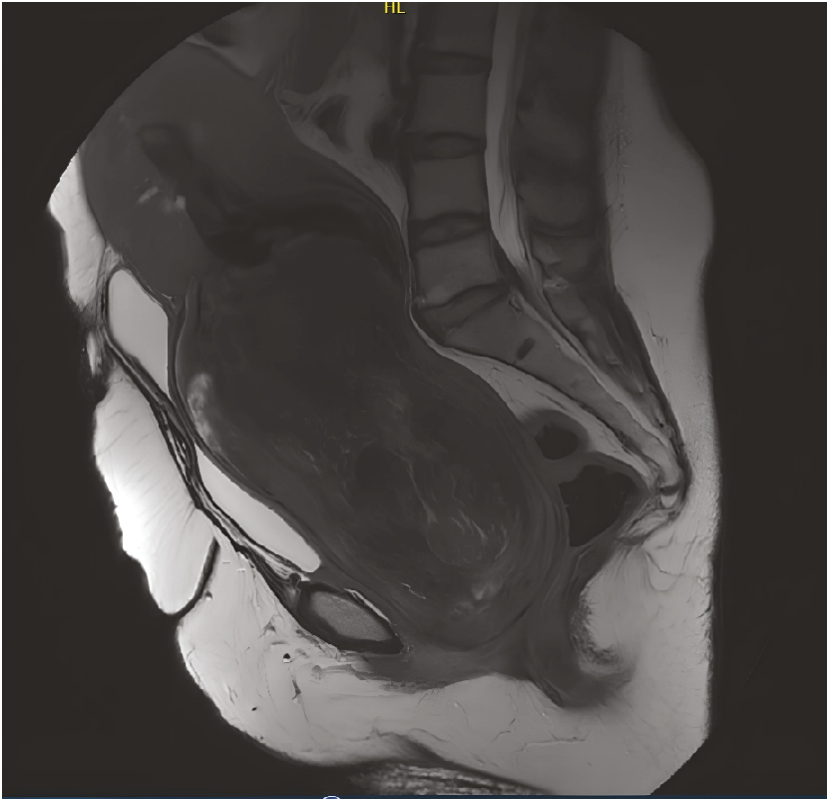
MRI showing pedunculated myoma in the vagina.
